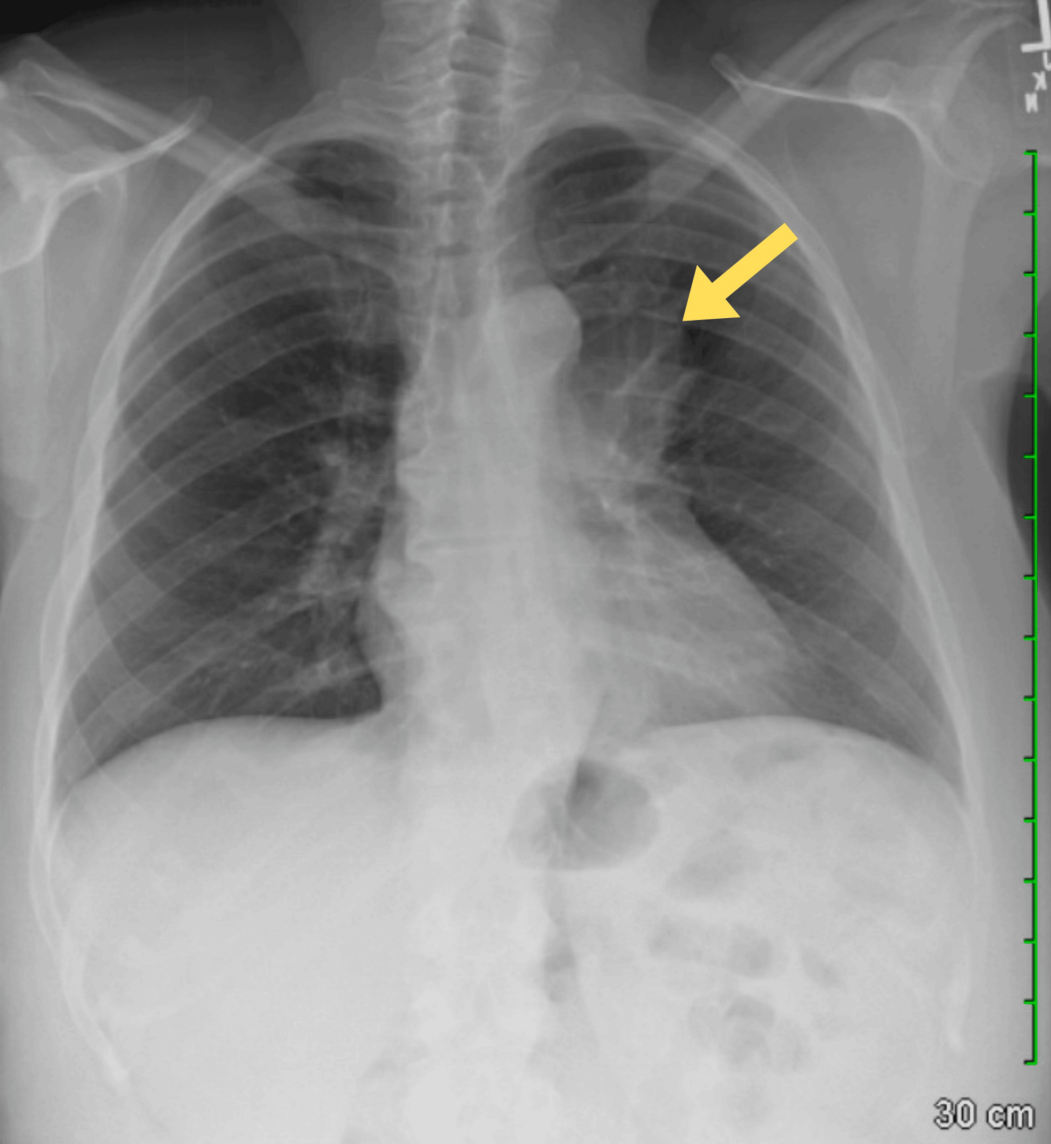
Chest X-ray showing a mediastinal mass (yellow arrow) present at the level of the aortic arch and aortopulmonary window. Bony structures display moderate degenerative changes.
